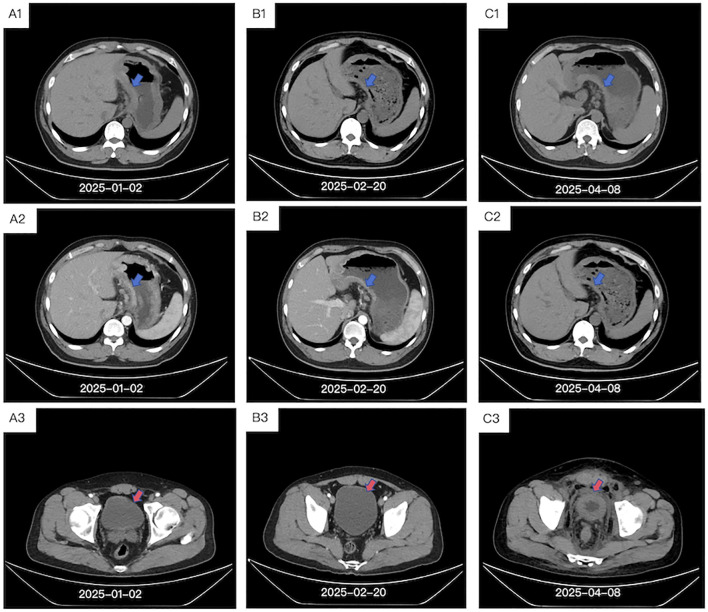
Computed tomography (CT) images during the disease course. (A1, A2) Baseline CT (January 2, 2025) showing diffuse gastric wall thickening (blue arrows): (A1) non-contrast scan; (A2) contrast-enhanced scan. (A3) No bladder wall thickening (red arrow). (B1, B2) After two cycles of treatment (February 20, 2025) demonstrating reduced gastric wall thickening (blue arrows): (B1) non-contrast scan; (B2) contrast-enhanced scan. (B3) No bladder wall thickening (red arrow). (C1, C2) At disease progression (April 8, 2025), both (C1) and (C2) are non-contrast scans due to impaired renal function: the gastric lesion shows no significant progression (blue arrows). (C3) Irregular bladder wall thickening consistent with metastatic involvement (red arrow).
